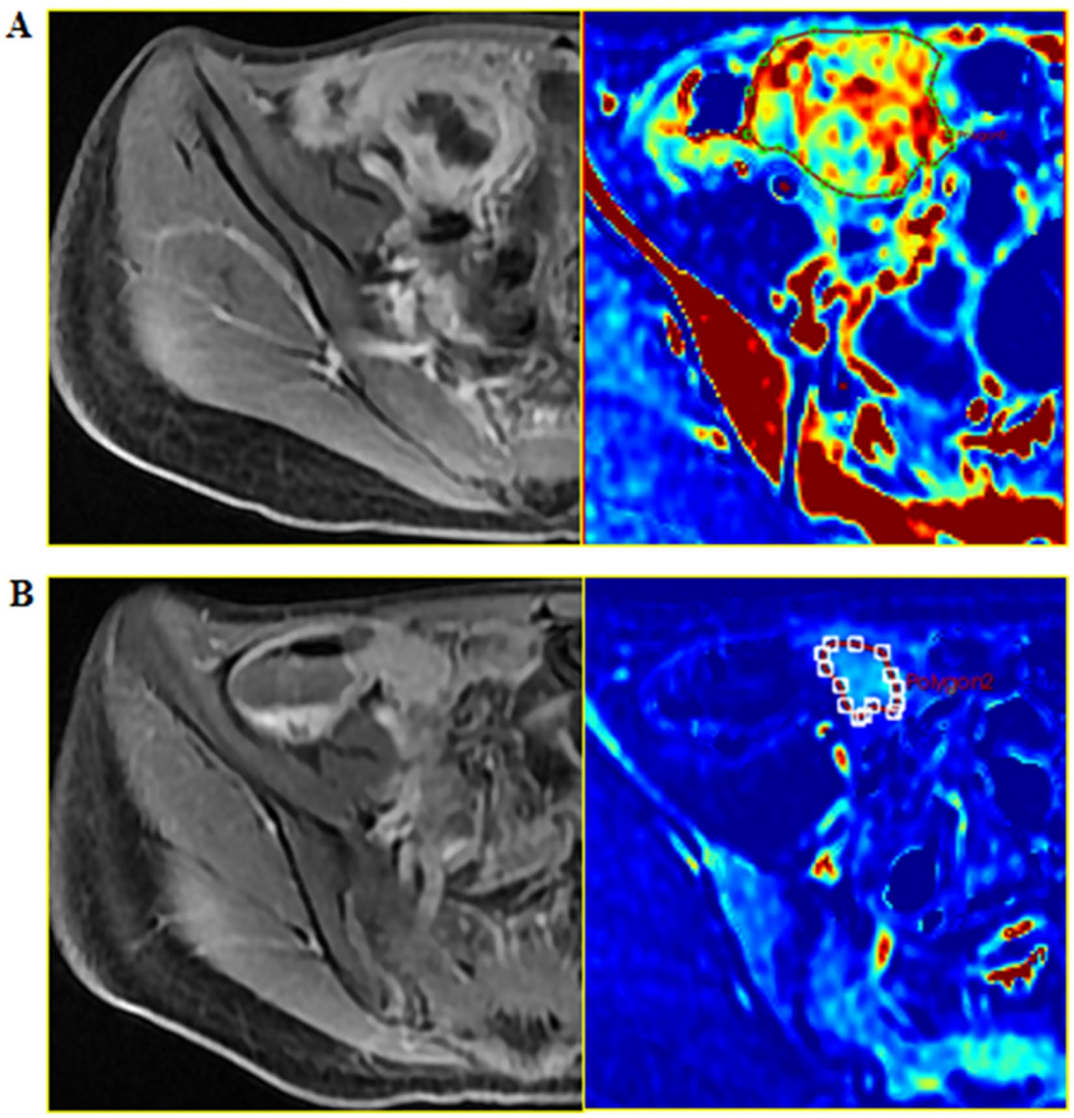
Dynamic contrast-enhanced MRI and diffusion-weighted MRI imaging improved the differentiation of CD and the related inflammatory mass. This case (patient 14) was diagnosed as a fistulizing mass at the terminal ileum ((A), before FMT) and was assessed as radiological improvement ((B), three months after FMT).
